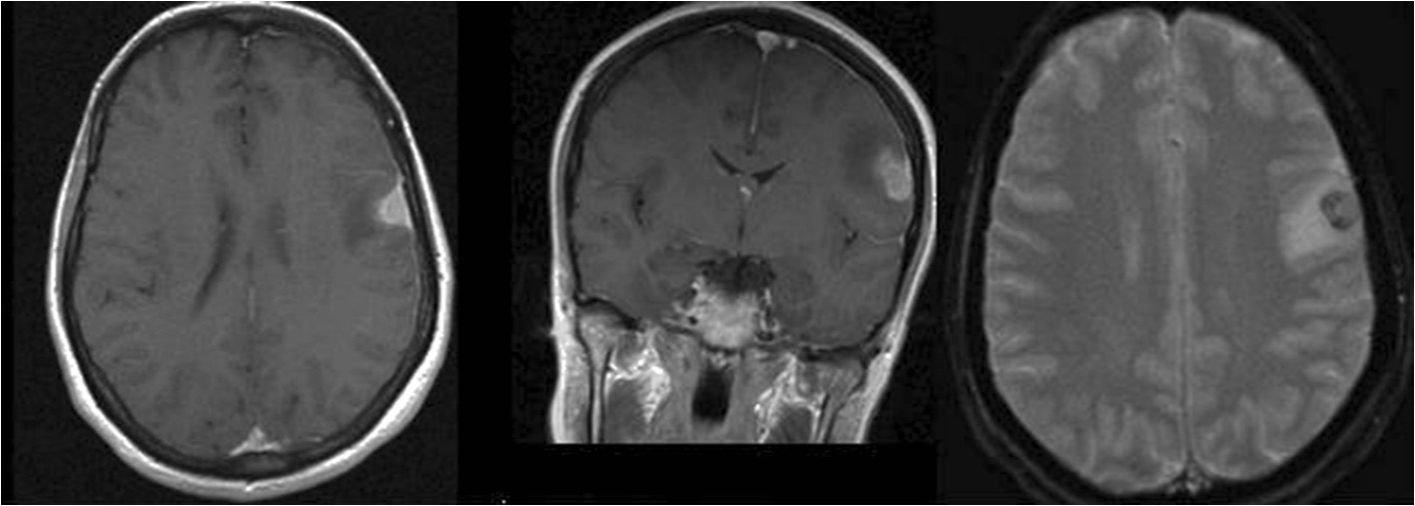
Magnetic resonance imaging demonstrating meningeal involvement and possible neoplastic disease. From left to right: Axial T1 image, coronal T1 image, and axial T2 image.
